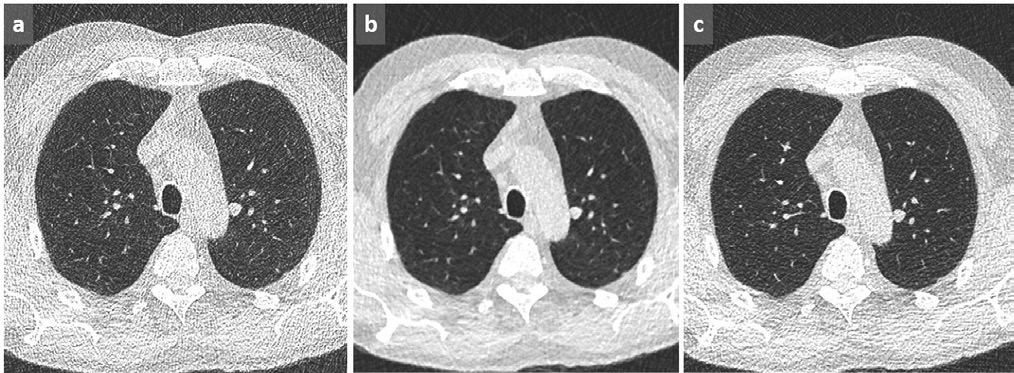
Lung CT acquisition with a CTDI at 0.45 mGy and DLP at 14 mGyxcm with lung (a), soft (b) and intermediate (c) kernel shows an optimized balance between image noise and spatial resolution with the intermediate filter at the same dose. CTDI, CT dose index; DLP, dose–length product.
